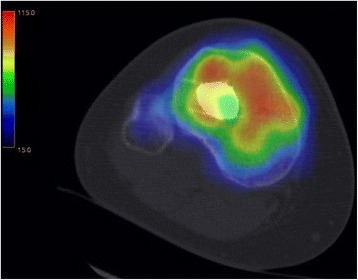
An axial view of a SPECT/CT corresponding to Figs. 6 and 7
